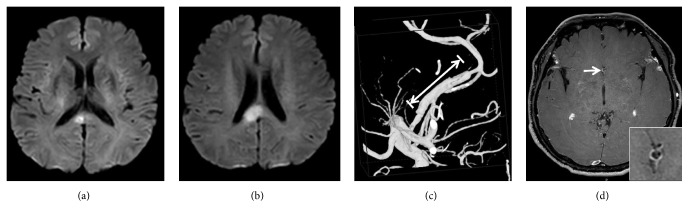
Male, 53 years, callosal infarction caused by anterior cerebral artery dissecting aneurysm.* Diffusion-weighted imaging (DWI) shows acute infarction in the right corpus callosum splenium (a-b). Three-dimensional digital subtraction angiography (DSA) confirms long segmental dilatation of the right anterior cerebral artery (ACA) (long white arrow) with distal concentric stenosis (c). Axial contrast-enhanced high-resolution magnetic resonance imaging (MRI) clearly reveals the enhanced wall of the ACA and an intima flap in the arterial lumen forming the "double-lumen sign" (white arrow) (d).
